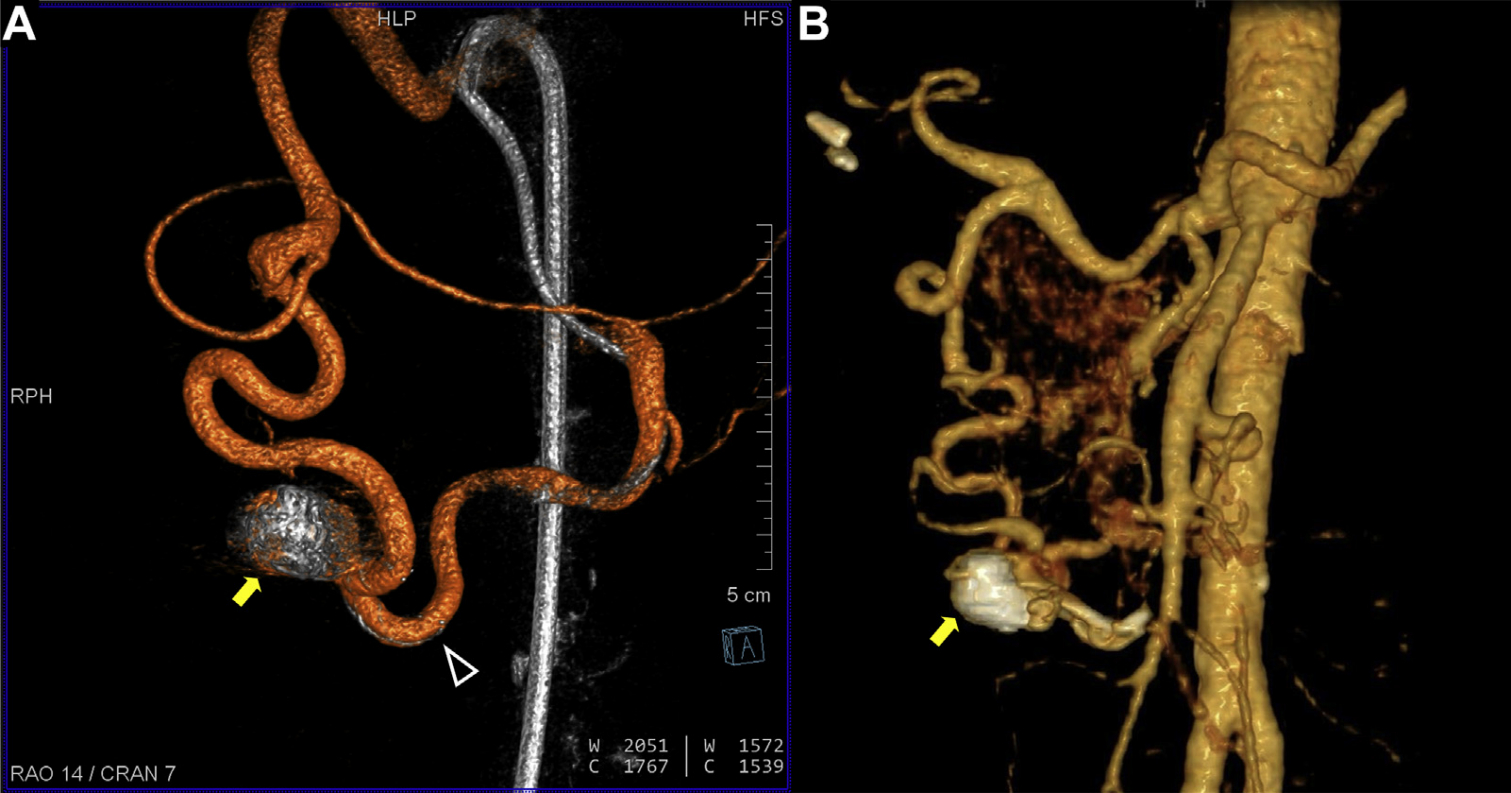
Comparison of three-dimensional (3D) volume rendered images of completion cone-beam computed tomography angiography (CBCTA) showing coils and low-profile visualized intraluminal support (LVIS) flow-diverter stent (A) and follow-up CTA (B) images showing patent pancreaticoduodenal arterial arcade with no aneurysmal contrast opacification (B). Proximal markers of LVIS flow-diverting stent were electronically marked with a *black arrowhead*.
